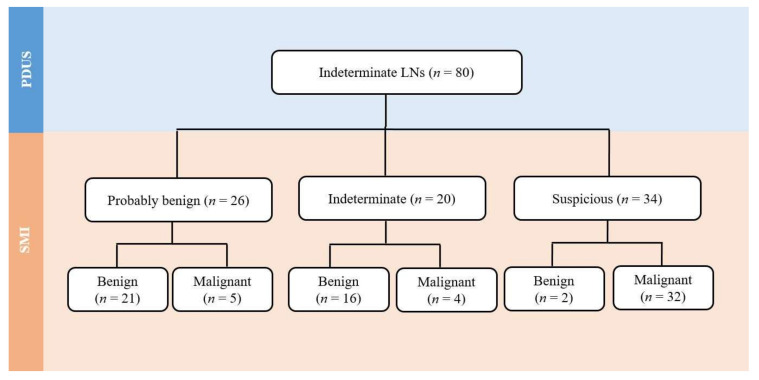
Reclassification of PDUS indeterminate LNs after the addition of SMI. LN—Lymph node, PDUS—Power Doppler ultrasound, SMI—Superb Microvascular Imaging.
